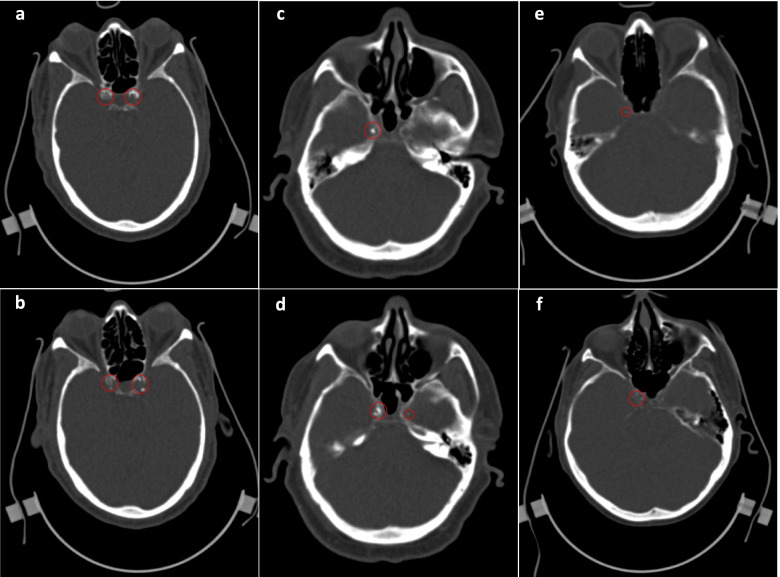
Internal carotid artery (ICA) calcification in low resolution CT scans for two participants. a, b Participant A demonstrates calcifications in the carotid siphon (total Agatston score: 1256). In participant B (c, d), calcifications are demonstrated in the petrous (c) and cavernous (d) segments of the left ICA (total Agatston score: 763). Mild ICA calcification is demonstrated in e–f in participant C with a total Agatston score of 150
